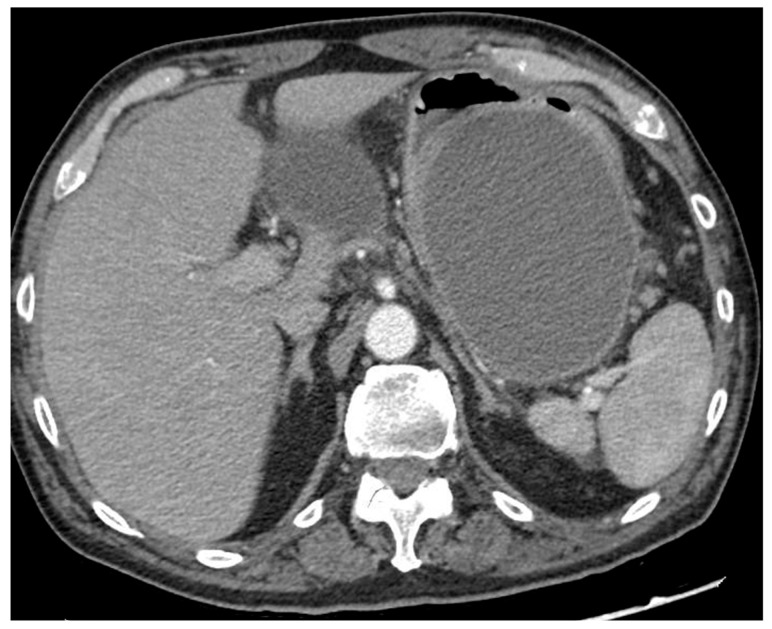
CT scan image of a large collection determining symptomatic gastric compression. CT, computed tomography.
